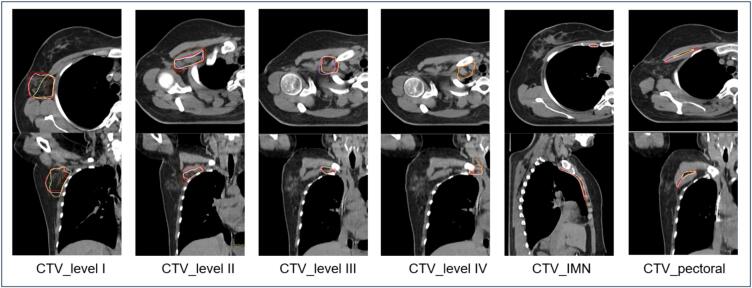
Axial and coronal CT images with resulting geometries of the CTVs for regional nodal irradiation for M1-3 (M1: pink, M2: brown, M3: yellow) and reference volume (red).
